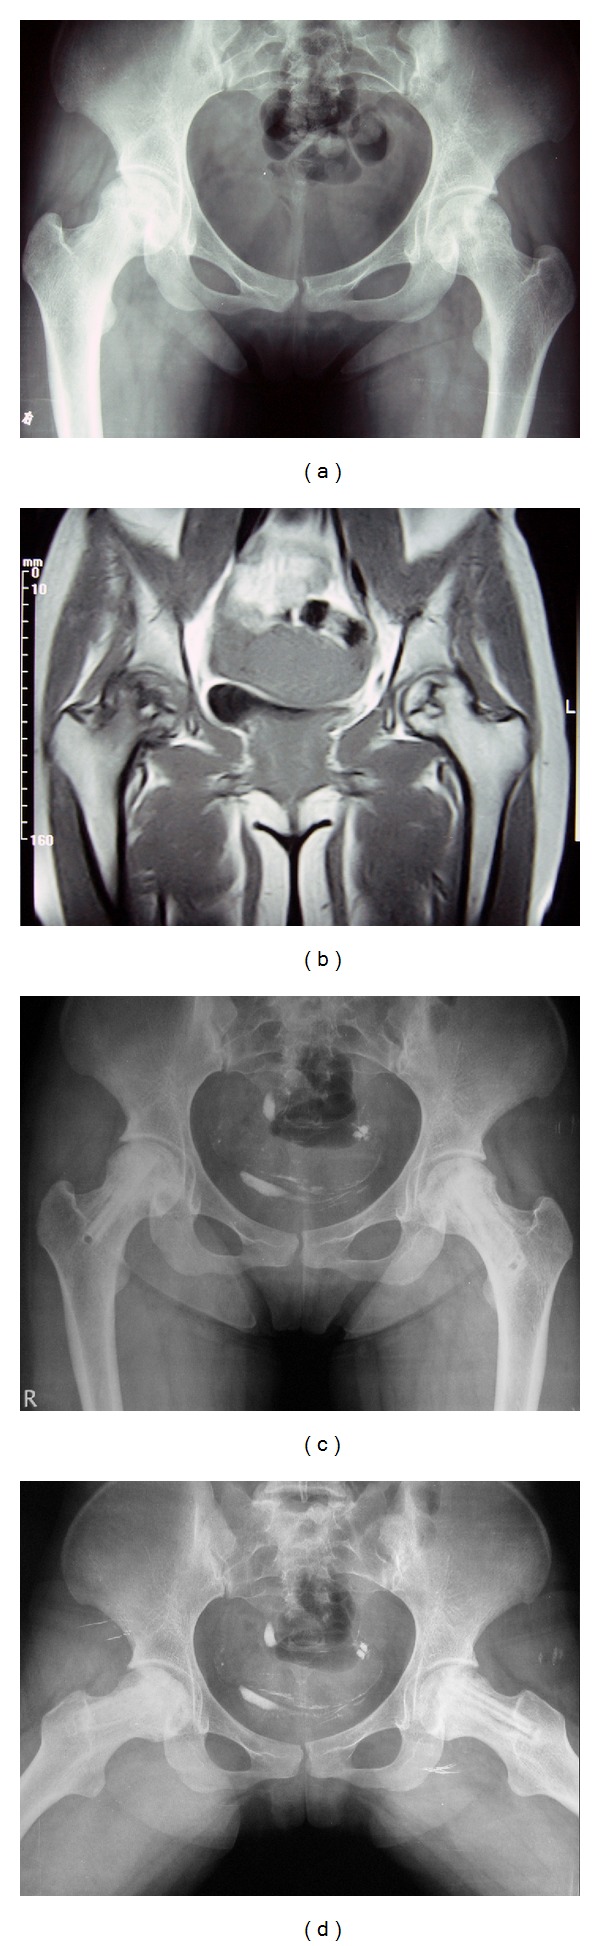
(a) Preoperative radiographs (anteroposterior position of the pelvis) of a 22-year-old woman with Lupus and stage II bilateral hip osteonecrosis. The preoperative Harris hip scores were 74 (right) and 85 (left). The patient received corticosteroid treatment at maintenance doses until the latest follow-up. (b) Preoperative MRI of this patient. (c) and (d) Postoperative radiographs (anteroposterior position and frog-leg position) taken three years after FVFG showed stability of the articular cartilage and femoral head. The postoperative Harris hip scores at the latest follow-up were 90 (right) and 96 (left).
